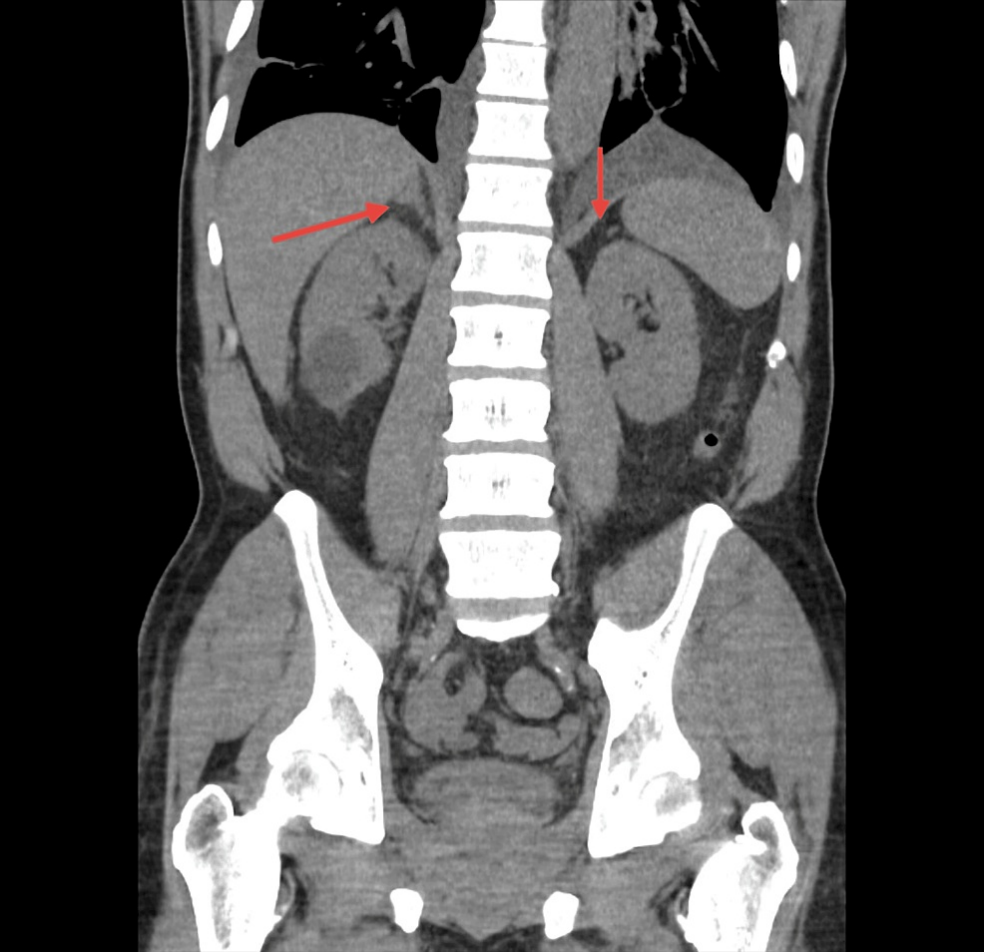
Non-contrast CT of the abdomen and pelvis does not portray any adrenal nodules.
